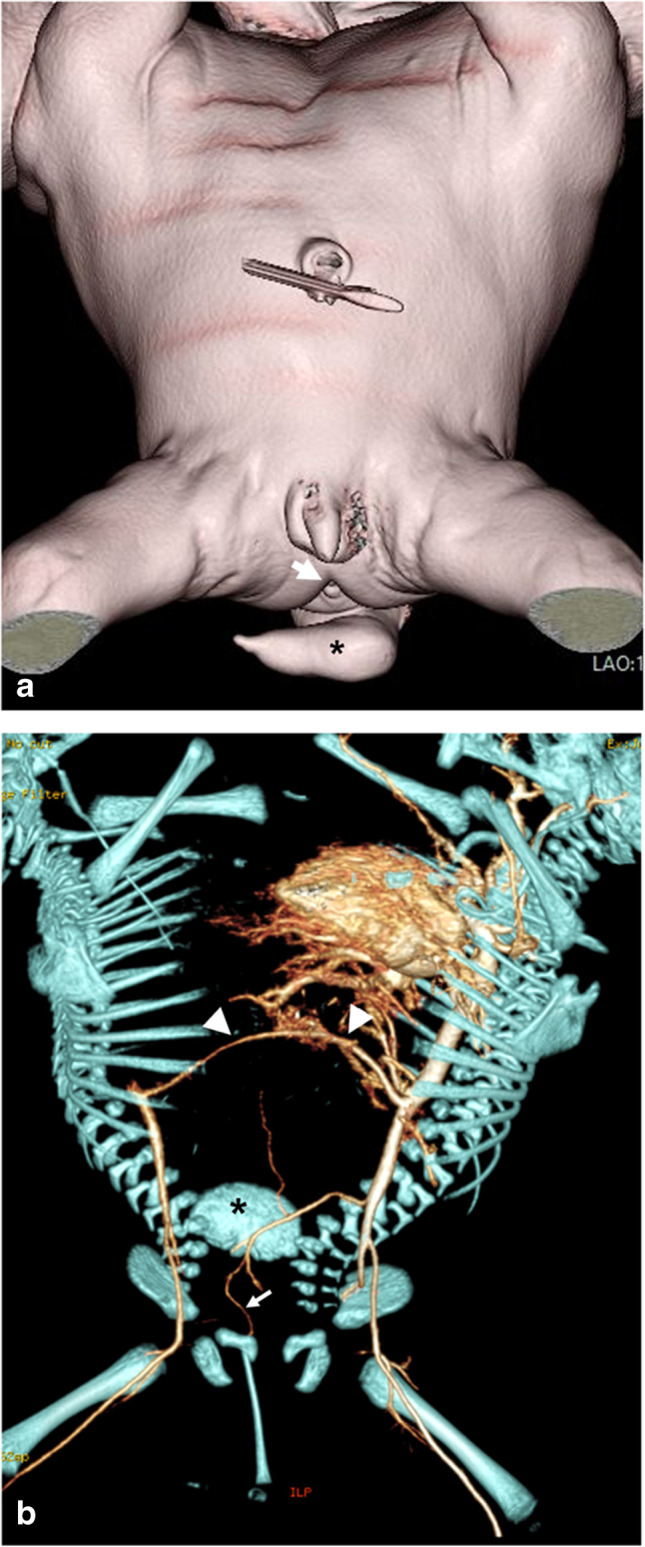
Computed tomography in 17-day-old ischiopagus tripus conjoined twin boys. a Surface-rendered image shows each baby to have one normal leg. There is a third deformed leg (*asterisk*) attached posteriorly to the conjoined pelvis. Note the single anal orifice (*arrow*) and single penis and scrotum. b 3-dimensional reconstruction of the skeleton and arterial tree following contrast injection of the left twin reveals a common iliac bone posteriorly (*asterisk*) and short bones of the deformed limb supplied by a small branch (*arrow*) arising from a hypoplastic right common iliac artery*.* A shared superior mesenteric artery (*arrowheads*) connects the aortas of both twins
